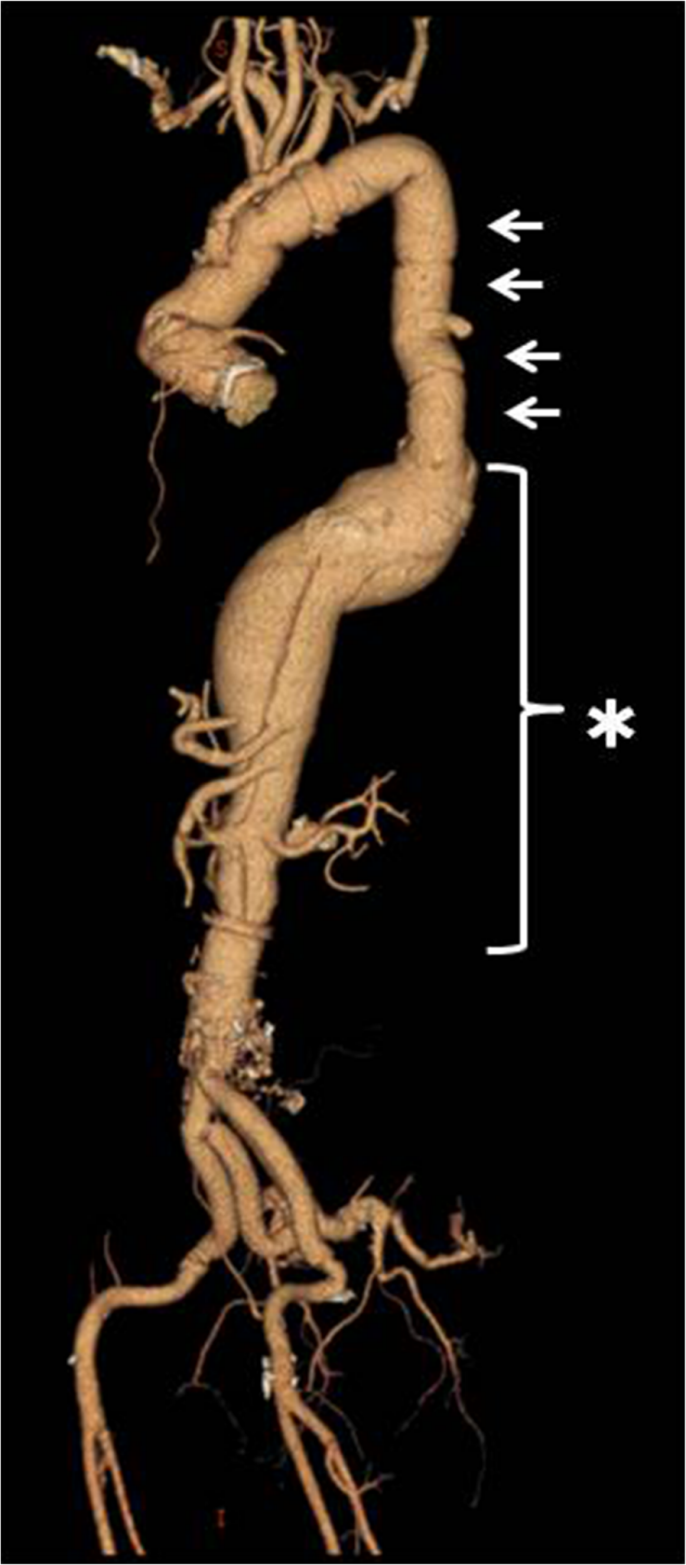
Preoperative three-dimensional CT showing a chronic dissectional thoracoabdominal aortic aneurysm from the descending aorta (Th7 level) to the infrarenal aorta (*asterisk*). The severe adhesions between the descending prosthetic graft and the *left* lung are predictable (*arrow*)
